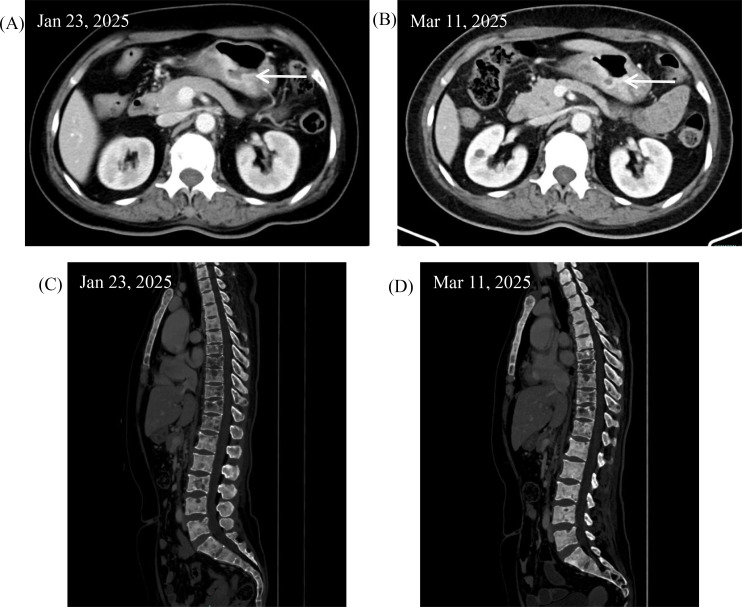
Follow-up CT scans indicating a stable disease (SD) after two and four cycles of first-line pemigatinib therapy. (A, B) Case 1 showing regression of gastric ulcerative lesions. (C, D) The lesion developed partial sclerotic fill-in of lytic lesions.
